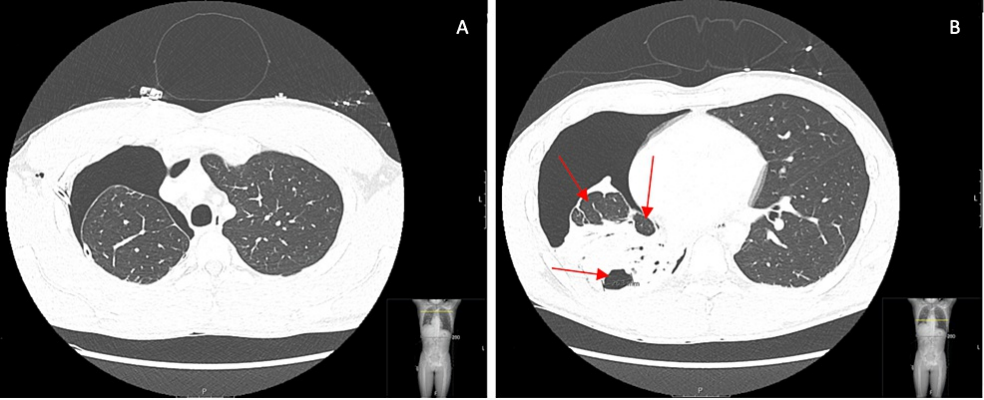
Initial chest CT with contrast of the upper (A) and lower (B) lungs in the axial view Observed is a large volume right pneumothorax with the collapse of the right lower lobe and subsegmental atelectatic changes of the right upper lobe. Red arrows point to persistent atelectasis of the right lower lobe with a parenchymal cystic lesion. Also observed was thickening and retraction of the visceral pleura with adjacent parenchymal fibrosis suggesting a trapped lung.
